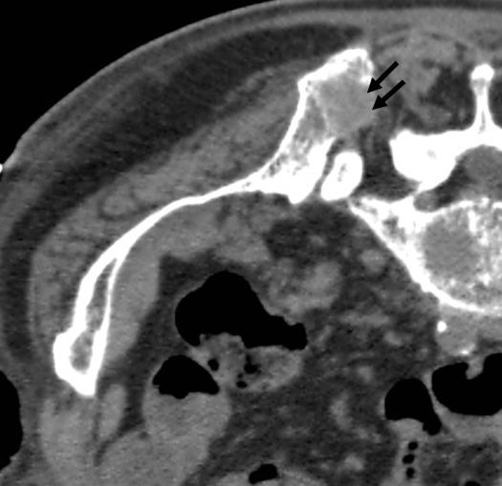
CT scan before the radiofrequency session. CT scan acquired with the patient in a prone position shows an osteolytic metastasis of 2.5 cm in the right back-upper ileum spine (black arrows).
